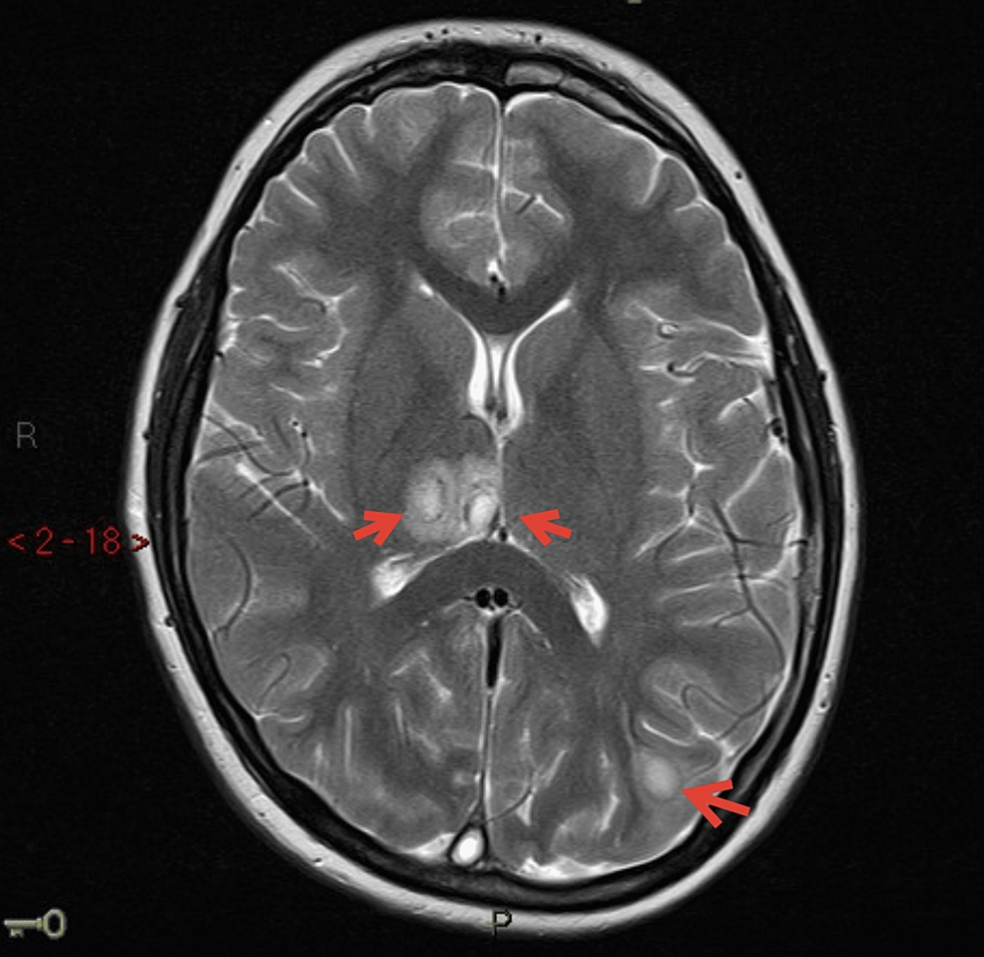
MRI of the brain with contrast. The red arrows indicate multiple ring-enhancing lesions in keeping with cerebral abscesses.
